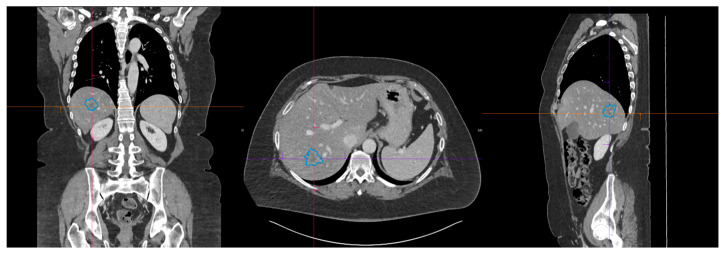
Portal venous phase CT displayed as multiplanar reconstructions: coronal (left), axial (center), and sagittal (right). The blue contour delineates the final 3D region of interest (ROI) of a representative hepatic lesion; contours are projected on each plane for visualization.
